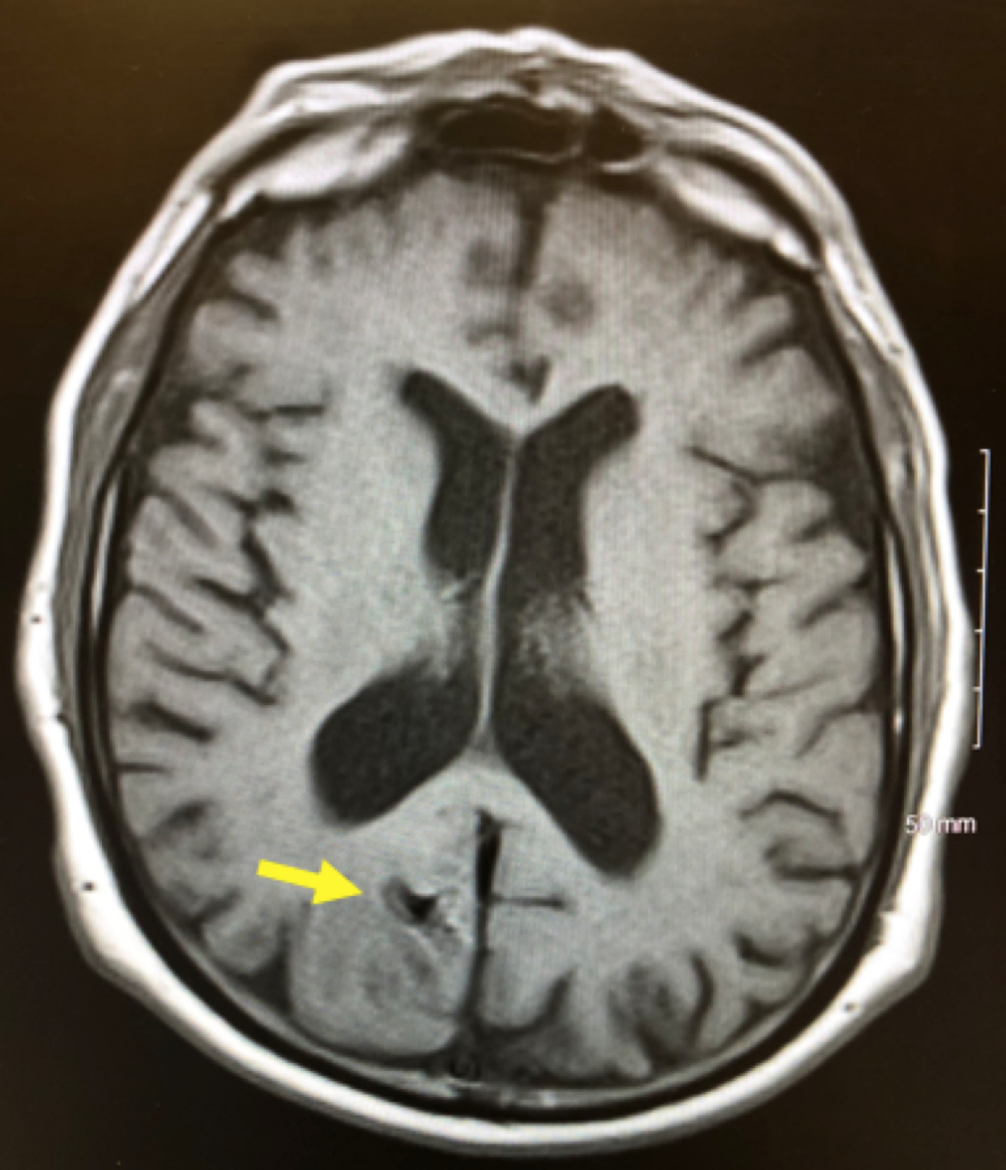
MRI brain with and without contrast demonstrating an enhancing mass in the right parieto-occipital medial cortex approximately 1.2 cm in diameter MRI: magnetic resonance imaging
